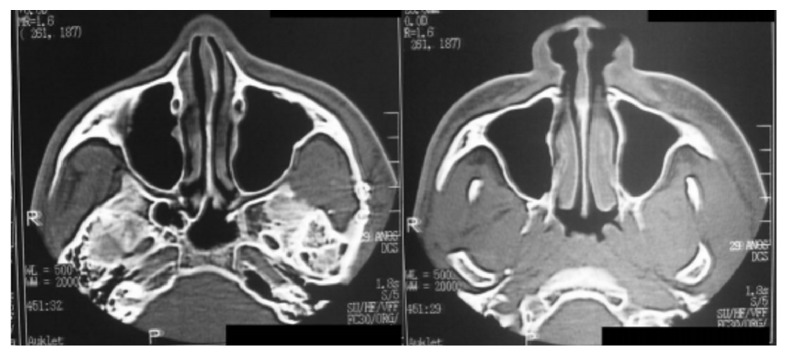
Postoperative computed tomography images.
